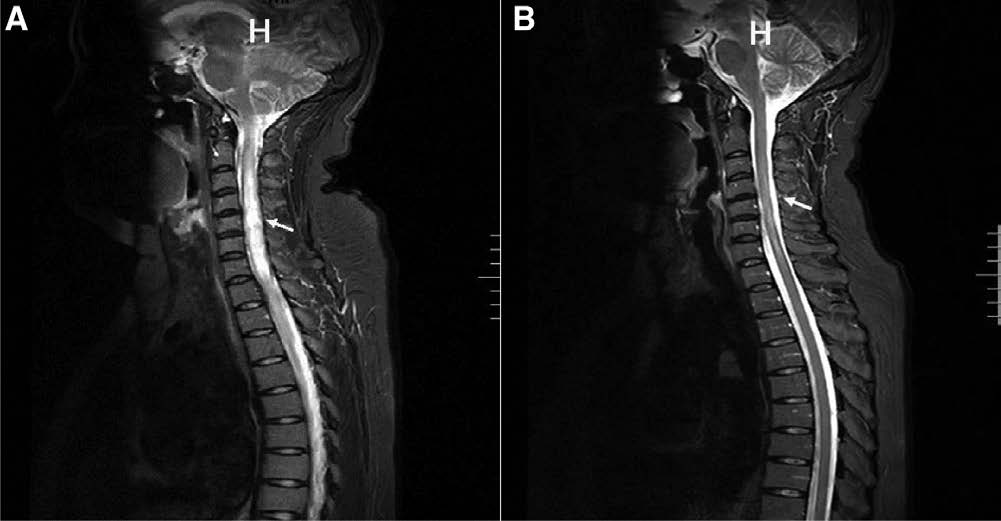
(A) Spine MRI on onset showed long strips and abnormal sheet signals from C2 to T3 with spinal cord swelling on T2WI (arrow). (B) Spine MRI on this admission showed abnormal signals from C3 to C7 and nearly complete resolution of spinal cord swelling on T2WI (arrow). H indicates head. MRI = magnetic resonance imaging, T1WI = T1-weighted images, T2WI = T2-weighted images.
